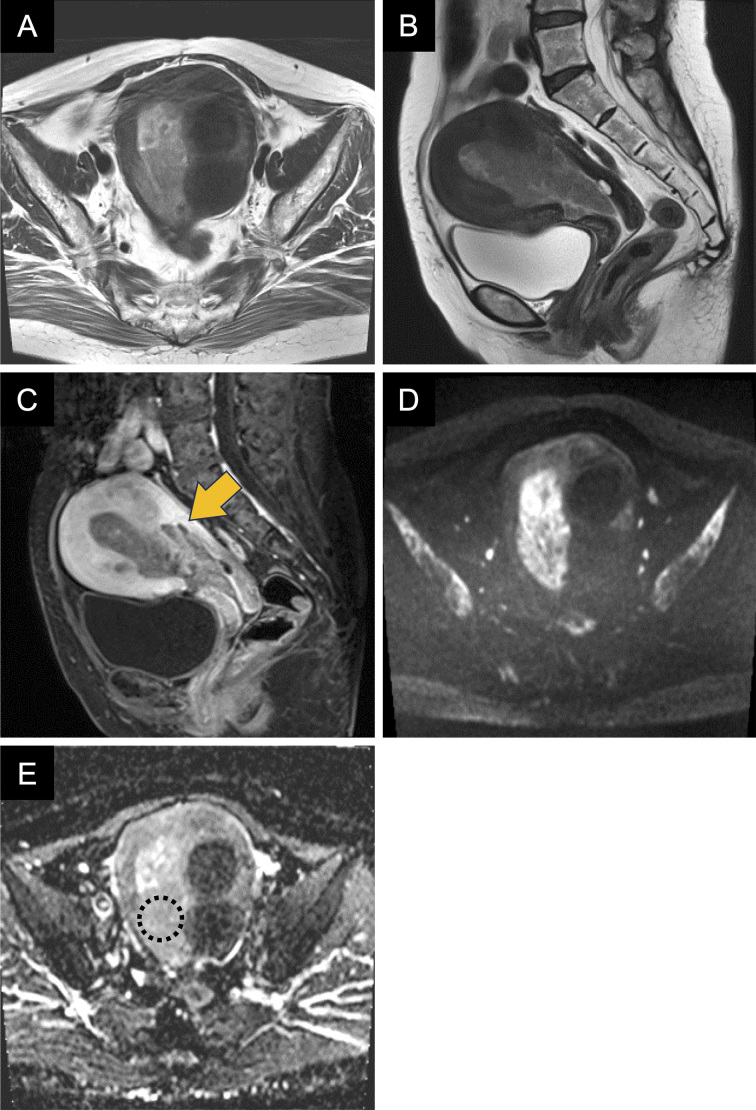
MRI findings of endometrial lesion. The lesion shows low signal intensity on the T2-weighted image compared to normal endometrium (A, B). There were no findings suggestive of adenomyosis. On contrast-enhanced T1-weighted image, the lesion demonstrates weak enhancement with suspected invasion into the posterior wall of the uterine corpus (C; arrow). While the lesion shows high signal intensity on DWI (D), the ADC value of 0.96 × 10^-3^ mm^2^/sec indicates no substantial diffusion restriction (E; dotted circle). Multiple uterine leiomyomas were observed. ADC: apparent diffusion coefficient; DWI: diffusion-weighted imaging; MRI: magnetic resonance imaging.
